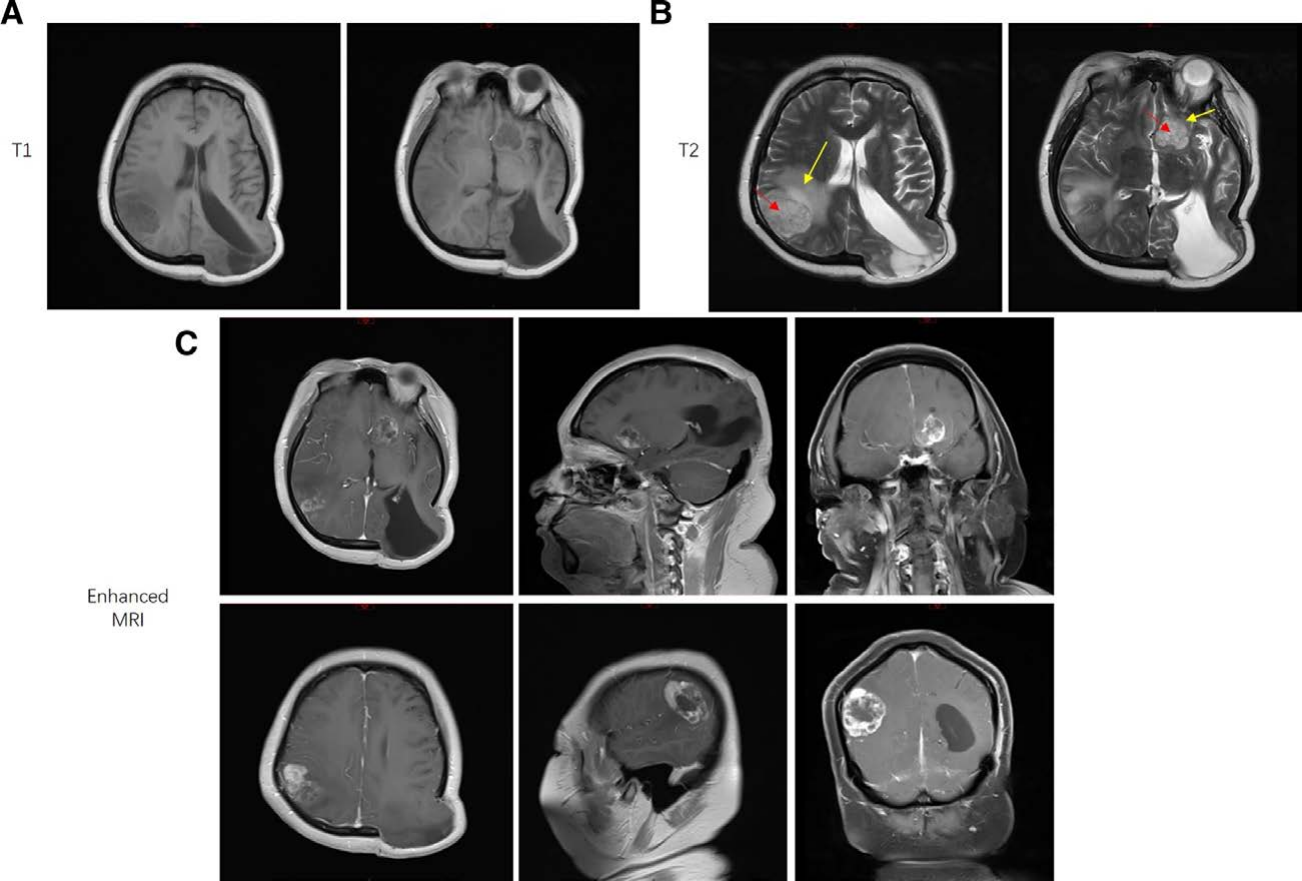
Brain magnetic resonance imaging (MRI) scan of the patient before surgery. A type of circular lesion was observed in the right parietal lobe with long signals on T1-weighted (A) and T2-weighted (B) images. The lesion was about 4 × 4 × 3 cm^3^ in size (red arrow) and accompanied by moderate edema of surrounding brain tissue (yellow arrow). Another type of circular lesion was deep in the left frontal lobe, with long signals on T1-weighted (A) and T2-weighted (B) images. The lesion was about 3 × 3 × 3 cm^3^ in size (red arrow) and accompanied by mild edema (yellow arrow). Enhanced MRI scanning showed that the lesions were unevenly and significantly enhanced (C).
